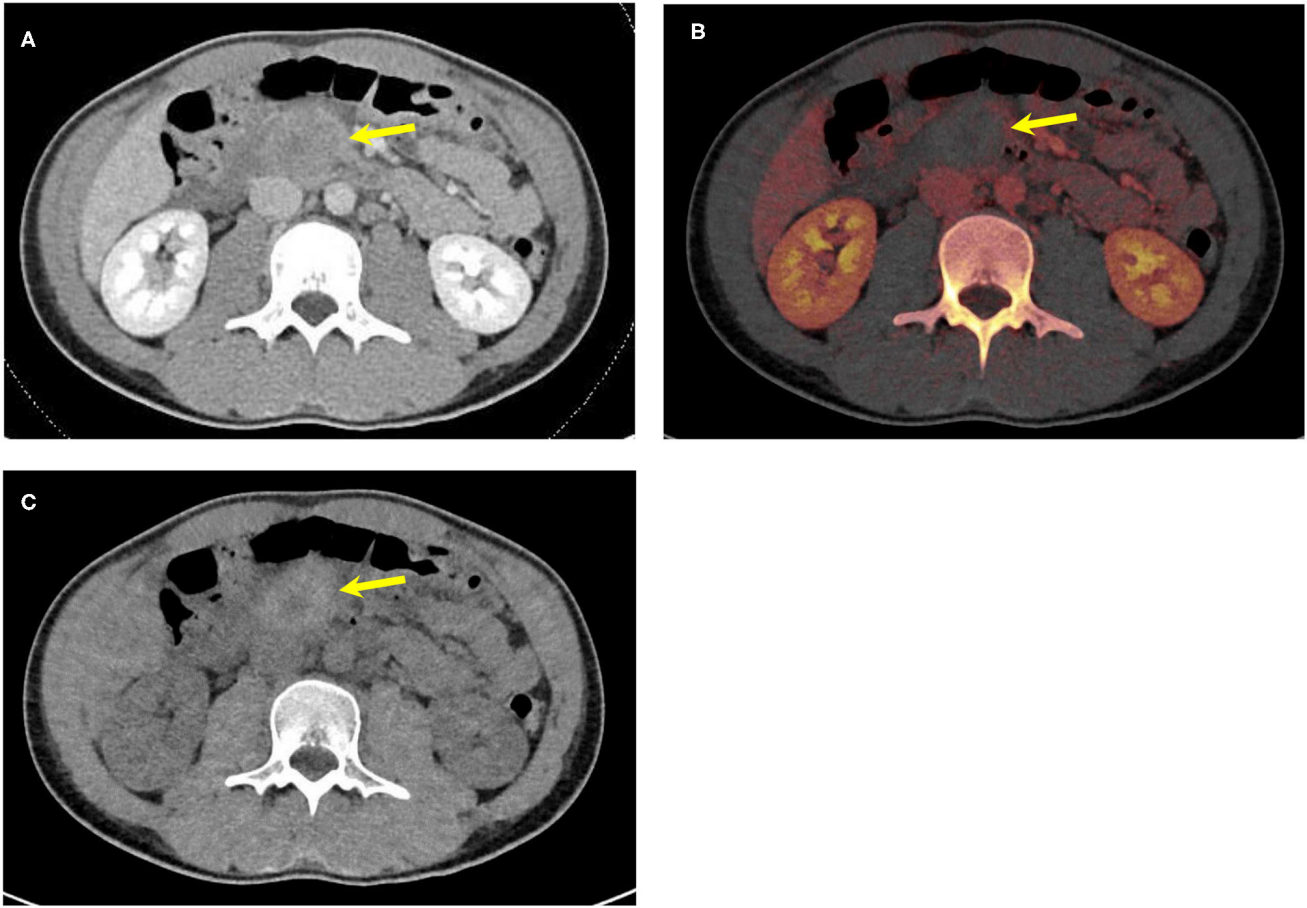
An 18-year-old male patient—hockey stick injury. (A) Conventional mixed DECT image demonstrates duodenal wall hematoma (yellow arrow). (B) Iodine overlay images increase the conspicuity of the duodenal hematoma by differentiating enhancing bowel from a non-enhancing duodenal hematoma (yellow arrow). (C) DECT VNC accentuates the hyperattenuating nature of the duodenal hematoma (yellow arrow). DECT, dual-energy CT; VNC, virtual non-contrast.
